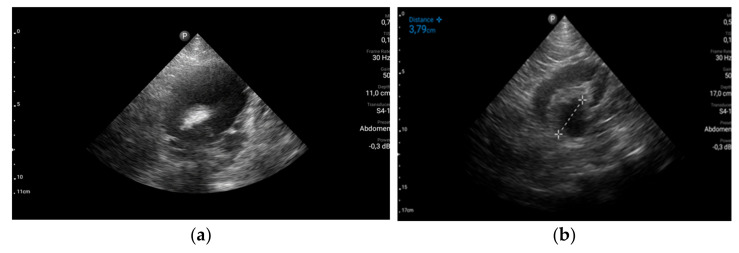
Abdominal ultrasound. (a) Hypogastric transversal section: urinary bladder obstructive stone, 3 cm in diameter and distended urinary bladder; (b) Right flank longitudinal section: third degree hydronephrosis of the right kidney.
